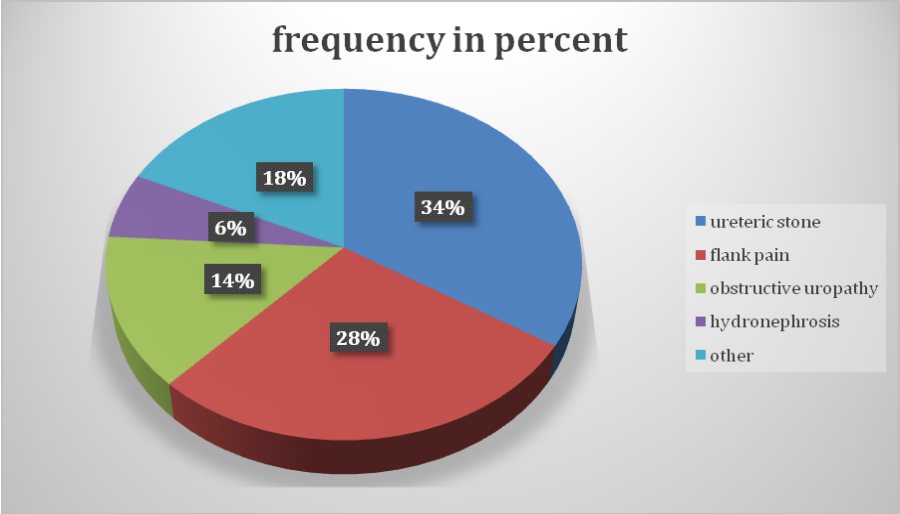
Pie chart showing common indications for the CT scans
